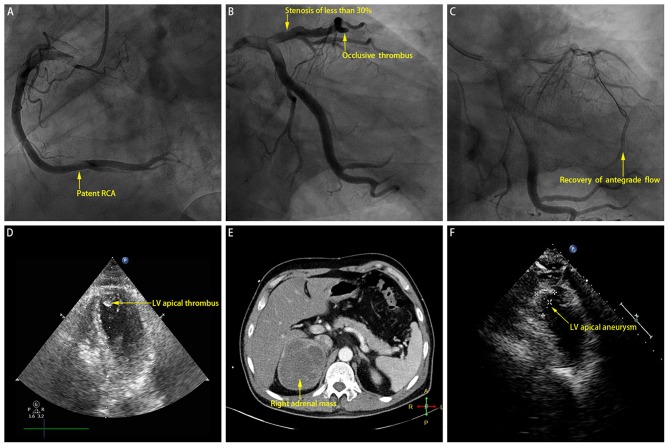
(A) Coronary angiogram revealing patent right coronary artery (RCA). (B) Coronary angiogram revealing minimal stenosis of <30% near proximal left anterior descending coronary artery (LAD) and occlusive thrombus in distal LAD. (C) Recovery of antegrade flow into distal LAD after manual thrombus aspiration. (D) Index echocardiogram revealing left ventricular (LV) apical thrombus. (E) Computed tomography scan revealing right adrenal mass which is of soft tissue attenuation with heterogeneous contrast enhancement. (F) Echocardiogram performed at the 2-month follow-up revealing left ventricular (LV) apical aneurysm.
